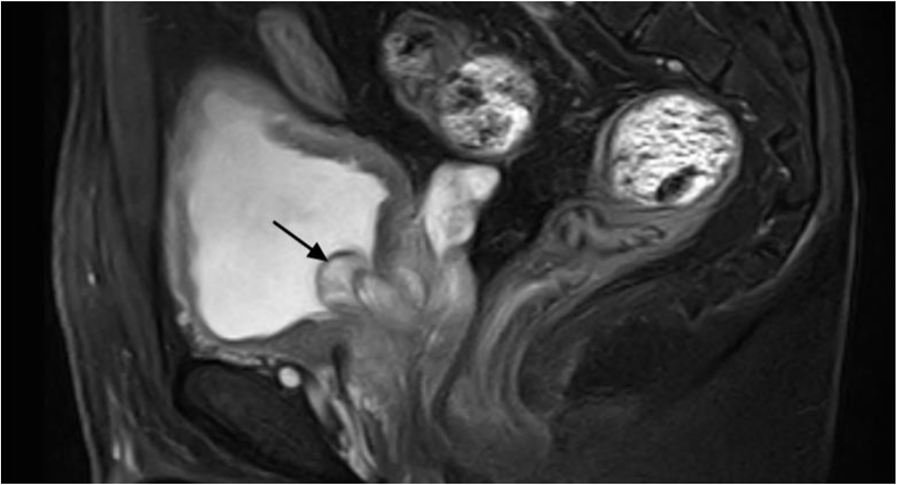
MRI—Sagittal section. T2 weighted phase. Bump of posterior urethral extruding to the bladder
